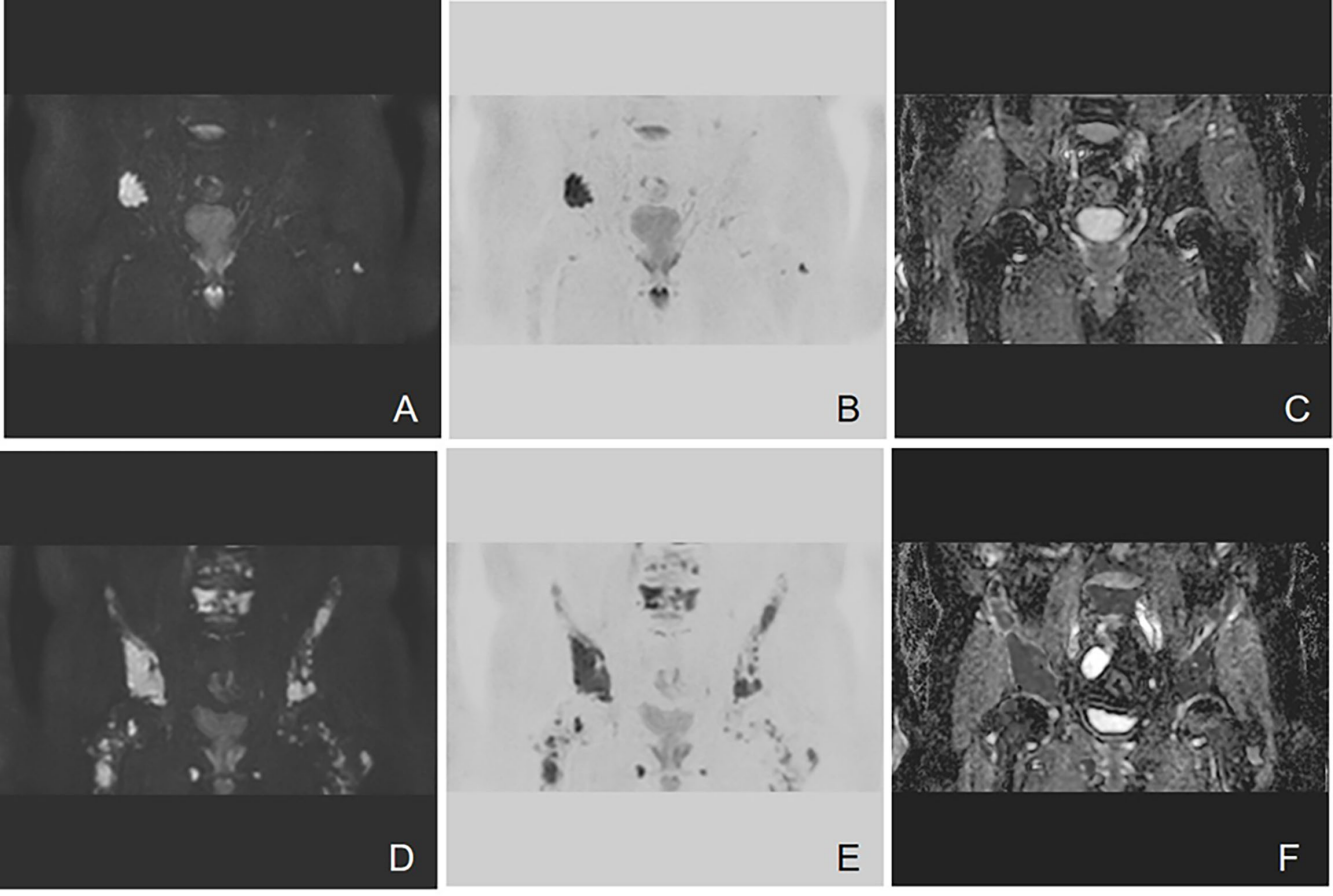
Example of DW-MRI image with treatment response of PD. A case of a 60-year-old man who was diagnosed with MM (type of IgG λ, RISS Stage II) is presented here. (A–C) Coronal images of DWI, inverted images, and ADC map at baseline visit. (D–F) Corresponding images of the same patient after three courses of induction chemotherapy (3 VCD). The treatment effect was evaluated as PD. Diffuse abnormal signal can be seen in the pelvis. It can be observed that the number of lesions increases and the scopes of most lesions expand. The mean ADC value of lesions in the scan range also increased from 0.743 × 10^−3^ to 0.881 × 10^−3^ mm^2^/s.
